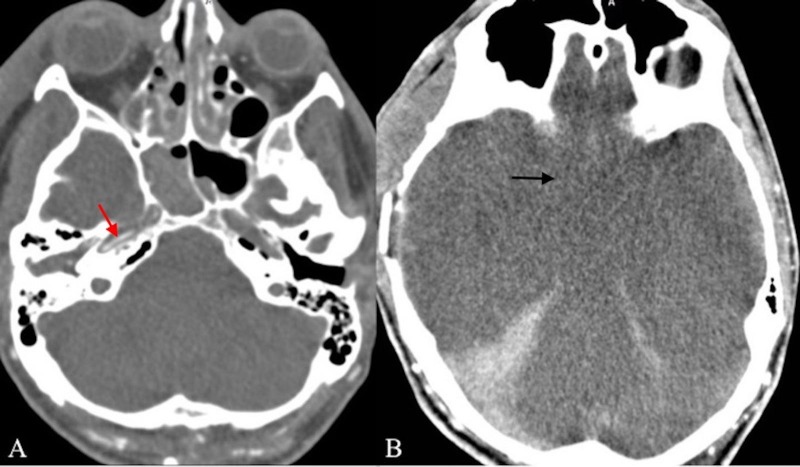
Axial CT angiography of intracranial arteries in two different levels. Images show flow in the petrous segment of internal carotid arteries (A, red arrow), which do not continue thereafter (B, black arrow). There is no flow in the posterior circulation.
